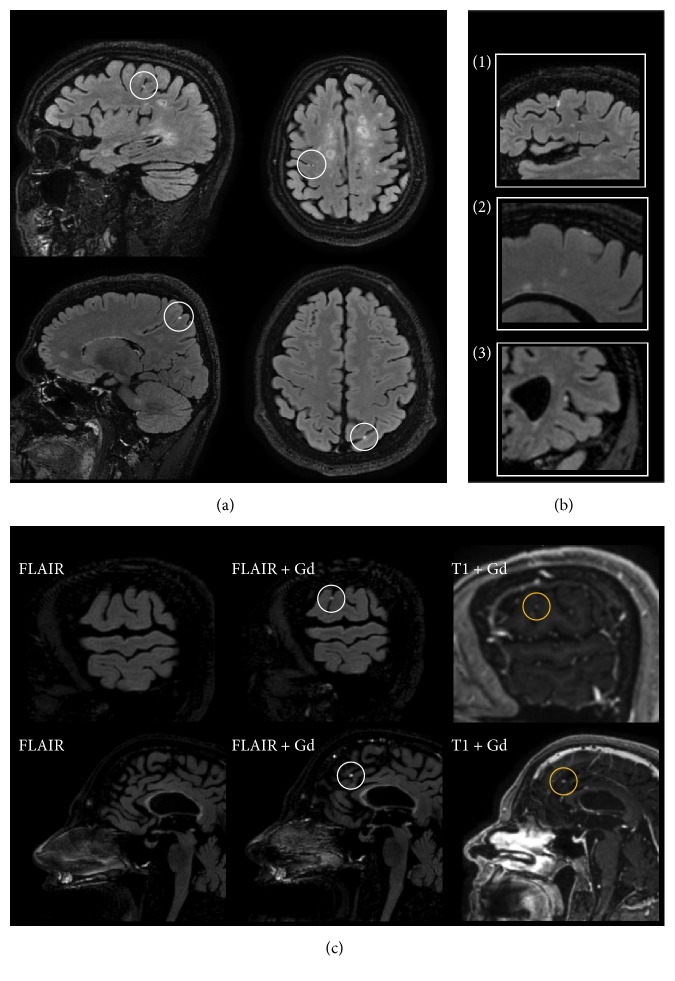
Main characteristics of LMCE. (a) Examples of localizations of LMCE on whole-brain images; (b) presentations of different types of LMCE foci: (1) linear, (2) plate-like, (3) nodular; (c) relationship between LMCE foci on precontrast FLAIR and postcontrast T1 and FLAIR.
